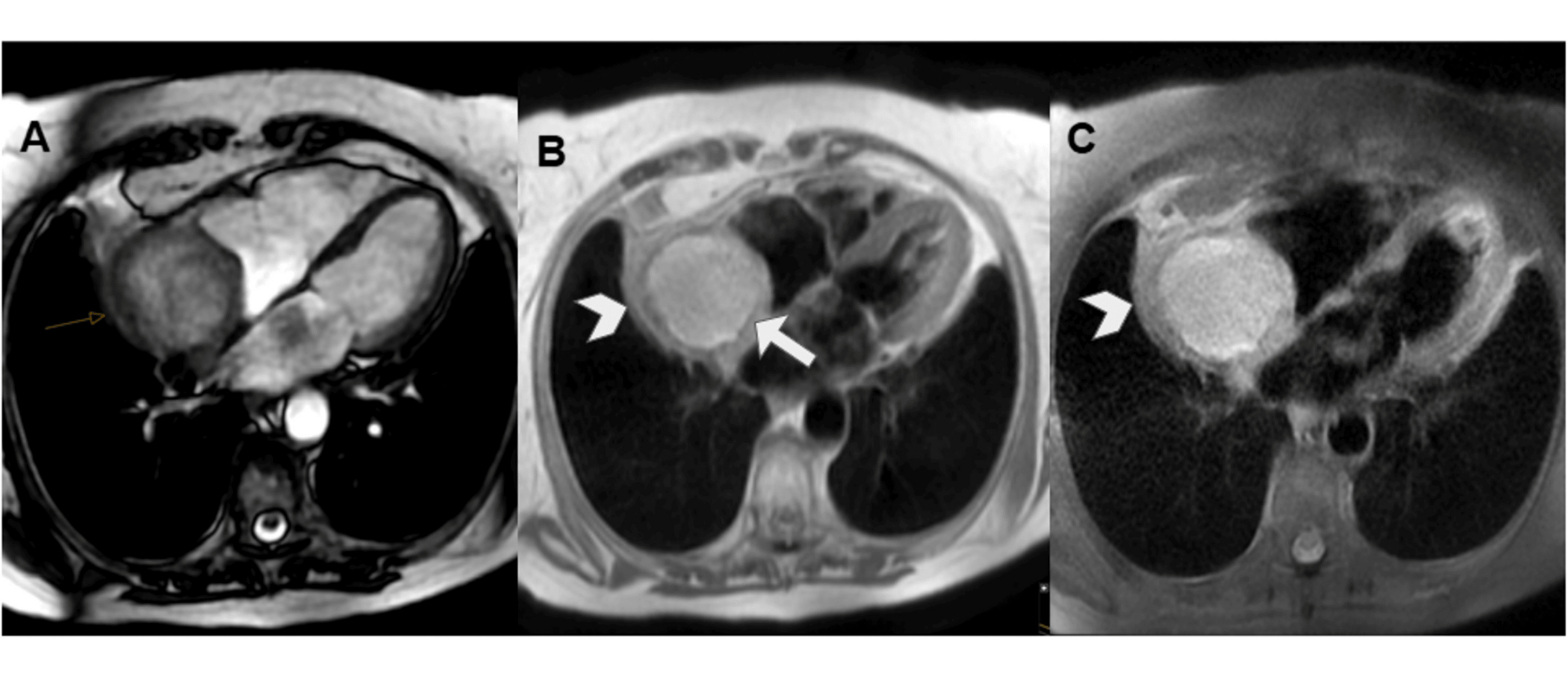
CMR imaging with steady-state free precession (A), T1-weighted turbo spin-echo (B), and T2-weighted turbo spin-echo with fat suppression sequences (C). These three sequences in an axial plane show a voluminous mass occupying the right atrium lumen. The mass showed heterogeneous signal with a central T1 hyperintense area, probably related to blood and/or proteinaceous content (arrow in B), and an irregular thickened peripheral wall that was slightly hypointense on T1 and slightly hyperintense on T2 (arrowhead in B and C). CMR: cardiac magnetic resonance.
